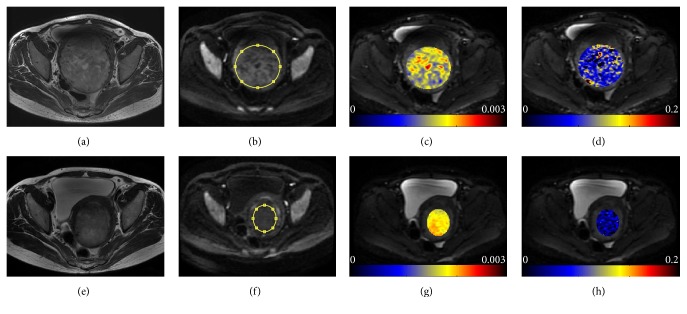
Images obtained in a 42-year-old woman treated with UAE for symptomatic fibroid before (a–d) and 6 months after embolization (e–h). (a, e) T2-weighted images demonstrate a submucosal fibroid which is oval shaped and heterogeneously hypointense. At 6-month follow-up, the fibroid exhibits remarkable reduction in size. (b, f) Placement of oval ROIs within the lesion on DWI. (c, g) ADC maps in the ROIs show increased diffusion properties at 6-month follow-up relative to baseline (1.85 × 10^−3 ^mm^2^/s versus 1.61 × 10^−3 ^mm^2^/s). (d, h) IVIM-derived perfusion fraction *f* maps in the ROIs show decreased microvascular perfusion/blood flow at 6-month follow-up relative to baseline (4.13% versus 7.98%).
